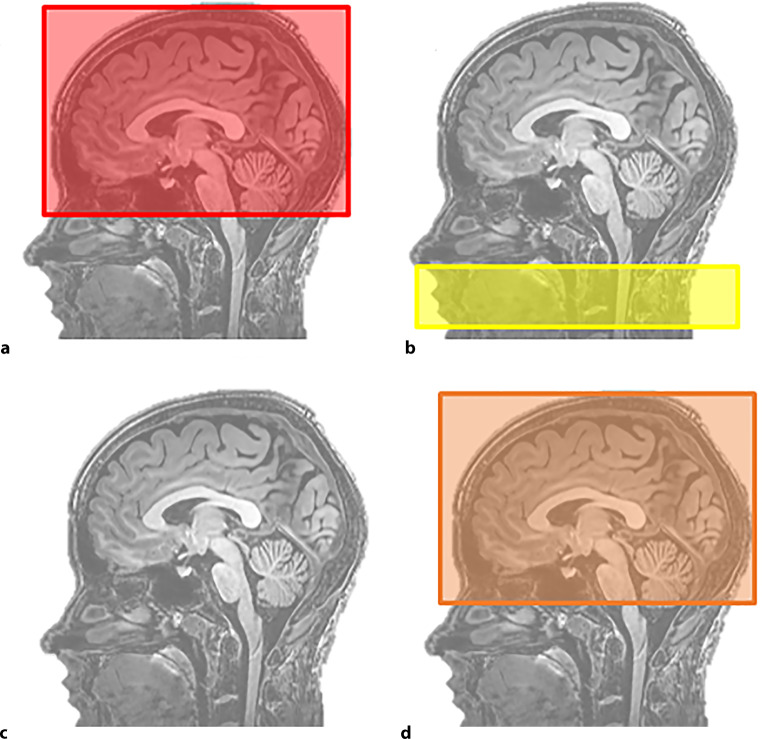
Arterial spin labelling magnetic resonance imaging acquisition. a Unlabelled scan, b endogenous tracer labelling in carotid artery, c labelled blood enters cerebral circulation and d labelled scan. Cerebral blood flow = labelled scan—unlabelled scan
